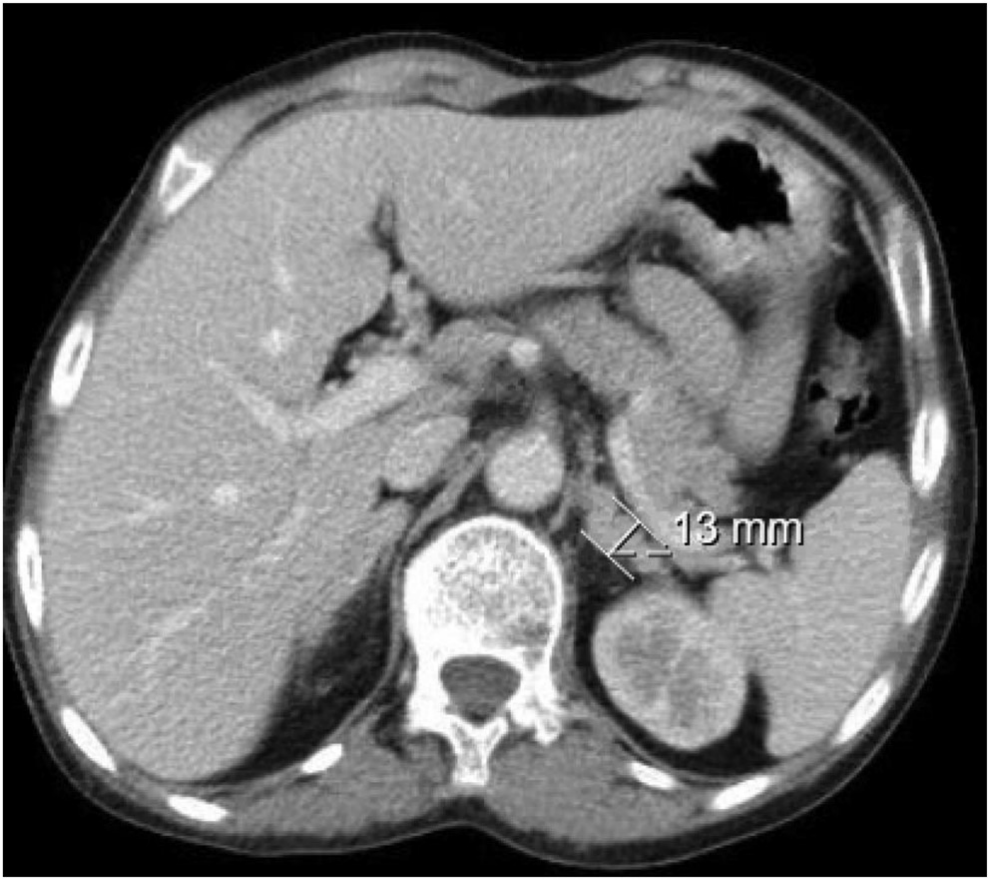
Abdominal CT scan showing adrenal bilateral hyperplasia – right width 11.5 mm, left width 12.9 mm.
